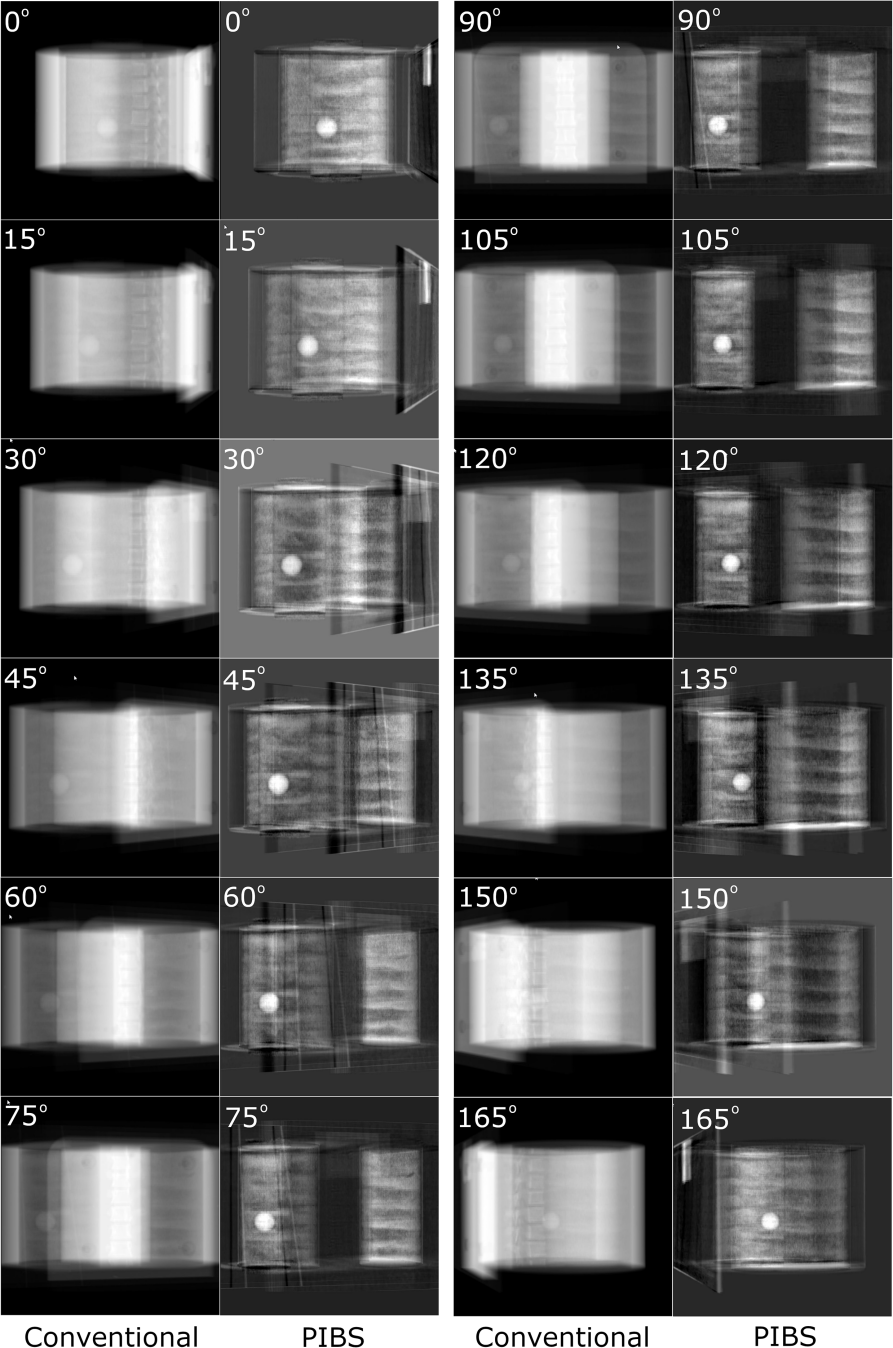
Comparison of conventional and PIBS X-ray images at projection angles of 0° to 179° at an interval of 15° for on-board imaging systems with 3D CBCT capabilities. Automatic window/level settings selected by 3D Slicer were used to display those images. PIBS X-ray was able to remove signal contributions from NE tissues and significantly enhance lung tumor visualization.
